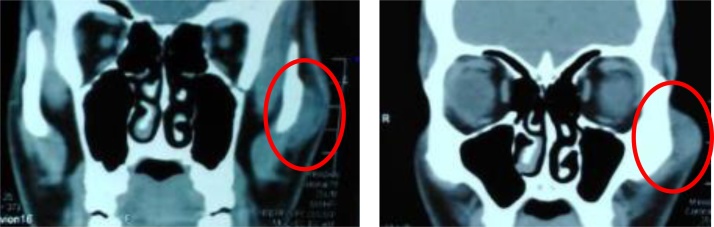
Coronal soft tissue CT scans obtained in 25 years-old Male: A circle shows heterogenous iso-density mass with discrete numerous low attenuation areas. A mass measuring 2.5 × 2 cm in size with well- contoured margin. which abutting on the left zygoma without radiographic signs of intra- bony involvement.
